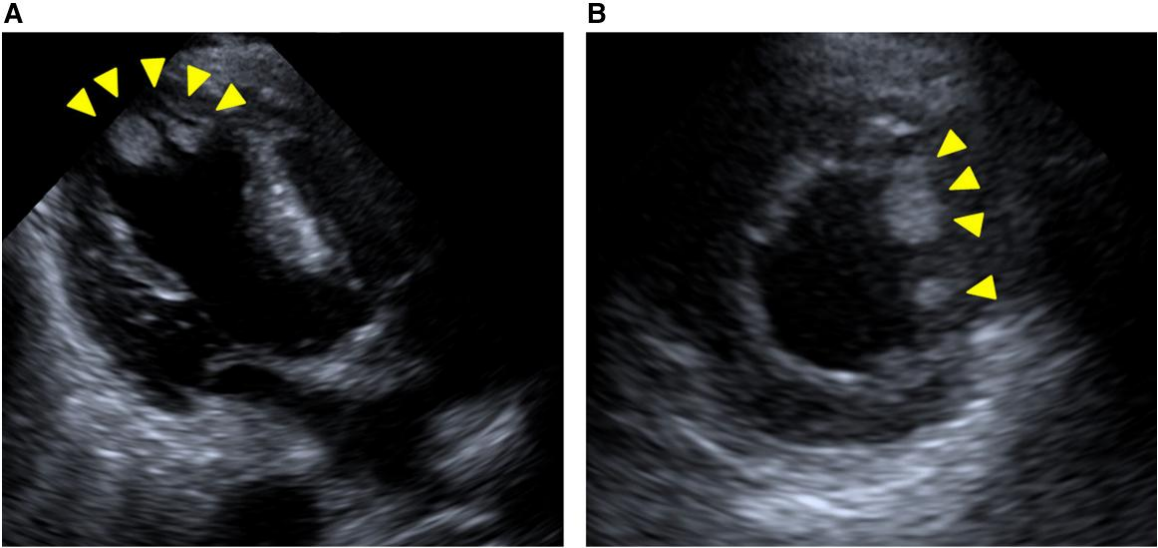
Transthoracic echocardiography showed multiple mobile thrombi in the apex of the left ventricle (arrowheads). (*A*) Apical three-chamber view. (*B*) Parasternal-short axis view at the apex.
